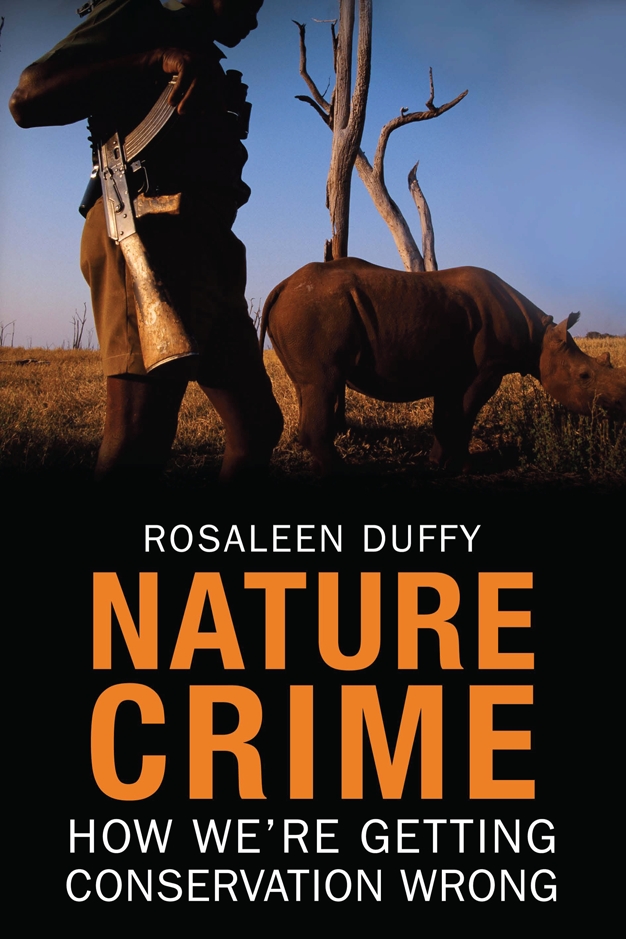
Duffy R (2010) Nature Crime: How We're Getting Conservation Wrong. New Haven, CT: Yale University Press. 288 p. ISBN 978-0300154344 (hardcover). US$42.00.
